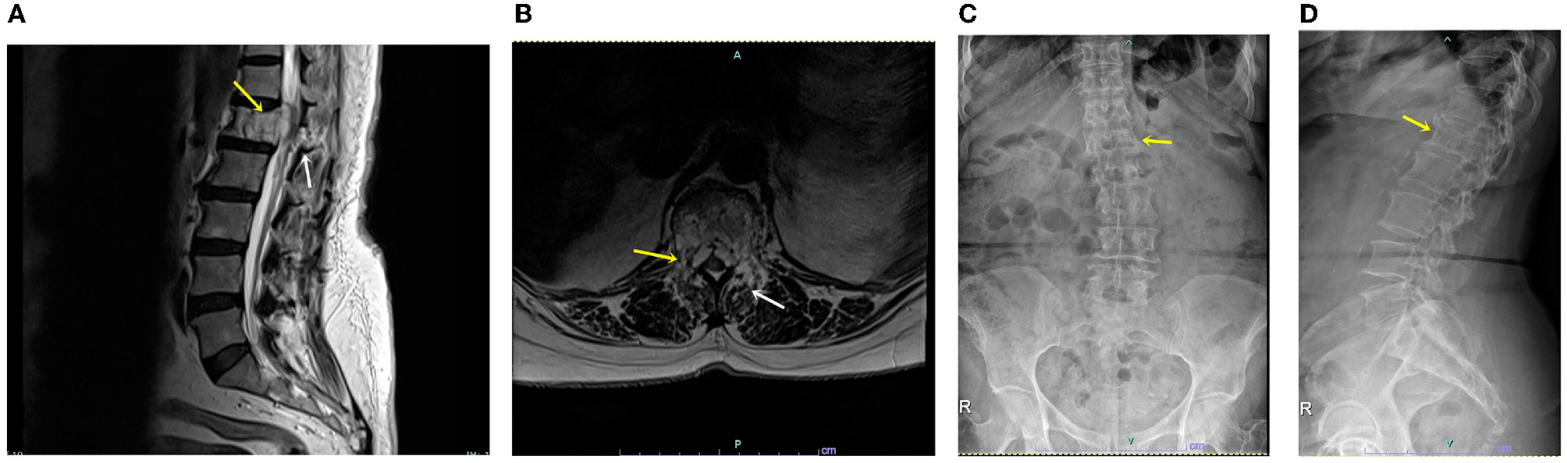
(A) Sagittal T2-weighted magnetic resonance image showing a lytic mass (yellow arrow) with spinal canal and posterior involvement (white arrow). (B) Axial T2-weighted magnetic resonance image showing the vertebral body, pedicle, and spinal mass (yellow arrow), as well as facet and laminar-mass extension (white arrow). (C) Plain anteroposterior radiograph showing decreased vertebral body height (yellow arrow). (D) Plain lateral radiograph showing heterogeneous density and decreased vertebral body height (yellow arrow).
